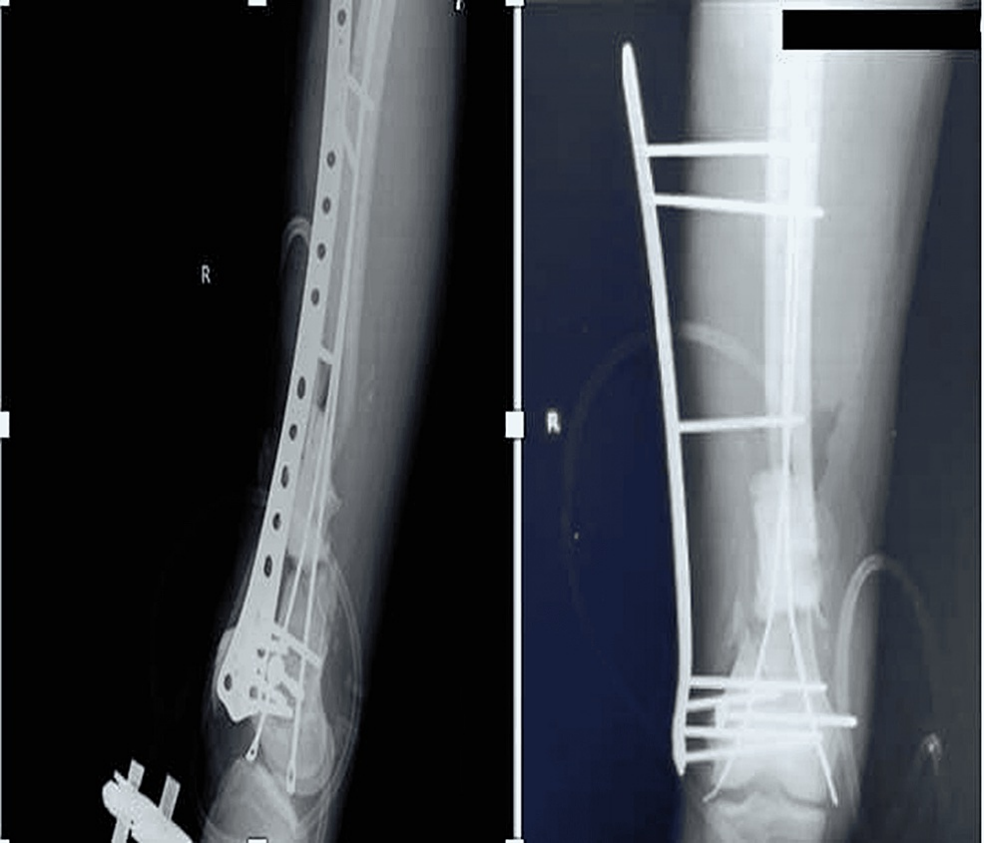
X-ray of right femur showing extracutaneous plate with cement spacer
